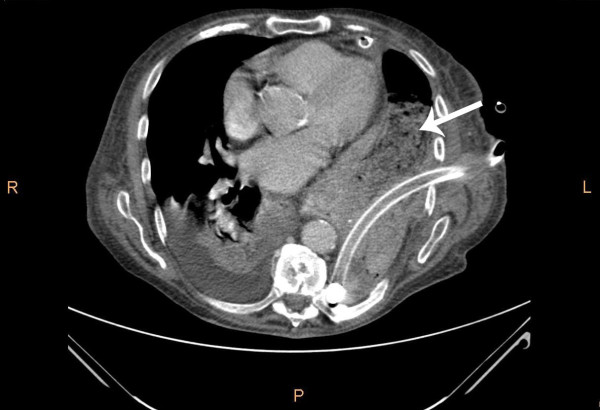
Computed tomography on the seventh day showing intrathoracic presence of bowel (colon transversum) with feces (arrow) and a basal chest tube.
